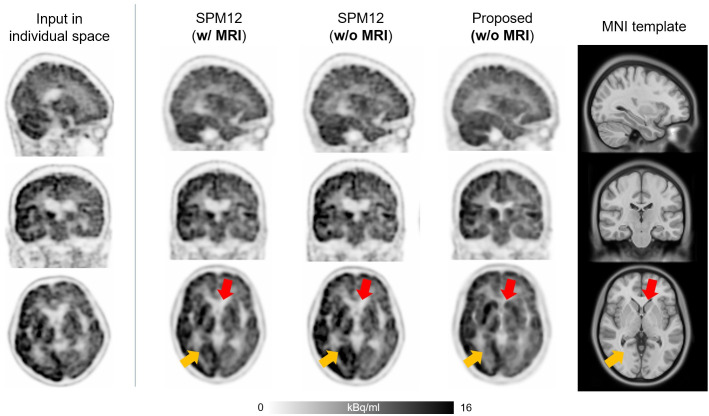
^18^F-FDG PET images of 72-y-old woman from internal dataset, exhibiting striatum atrophy. Although SPM did not perfectly reshape region (red arrows), proposed method showed significant improvement, also outperforming SPM in occipital cortex (indicated by yellow arrows).
